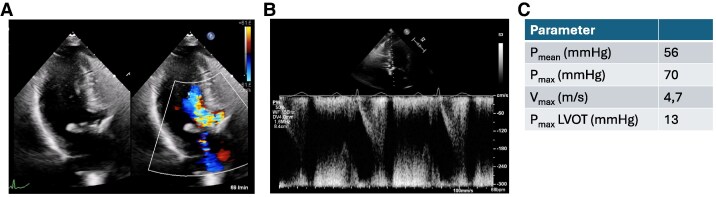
Transthoracic echocardiography before transcatheter aortic valve implantation treatment. (*A*) Apical three-chamber view of the aortic valve in systole showing severe flow acceleration and (*B*) pulse wave Doppler in the left ventricular outflow tract. (*C*) Doppler over the aortic valve.
